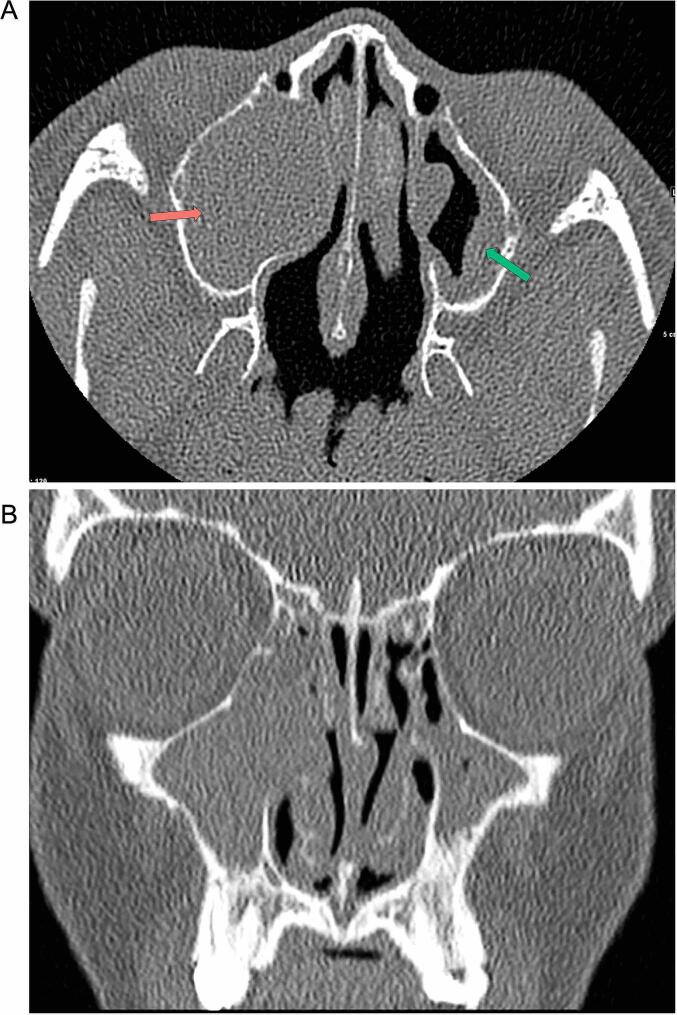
Sinus CT at 11.3y reveals pansinusitis disease most pronounced in the maxillary sinus. (A) Axial view is notable for mucocele causing complete opacification of the right maxillary sinus (red arrow) and mucosal thickening (green arrow). (B) Coronal view demonstrates patchy mucosal thickening throughout the ethmoid sinuses. For interpretation of the references to color in this figure legend, the reader is referred to the web version of this article.
